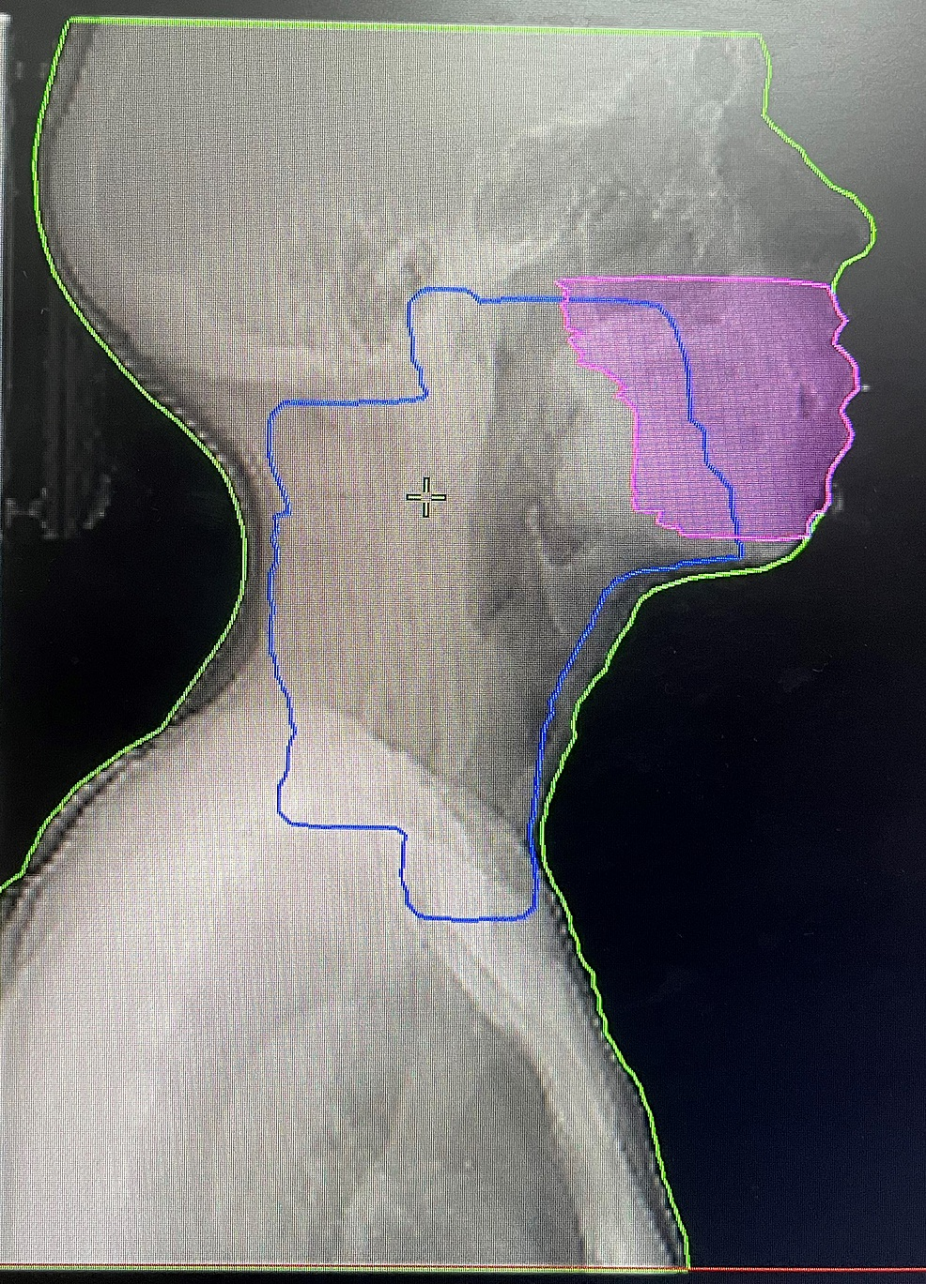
Digitally reconstructed radiograph in the lateral view shows PTV (blue) and pseudo-OAR (pink) PTV, planning target volume; OAR, organ at risk
